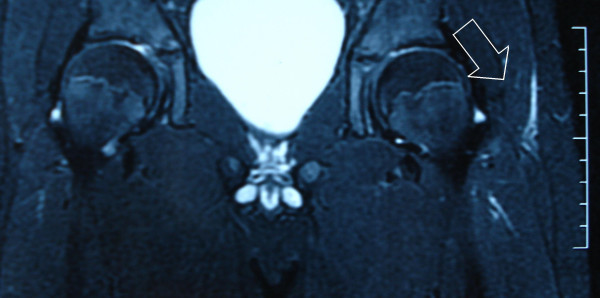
Control magnetic resonance imaging of the left hip (5 days after antibiotherapy) showing a significant decrease in the extent of involvement (arrow).
